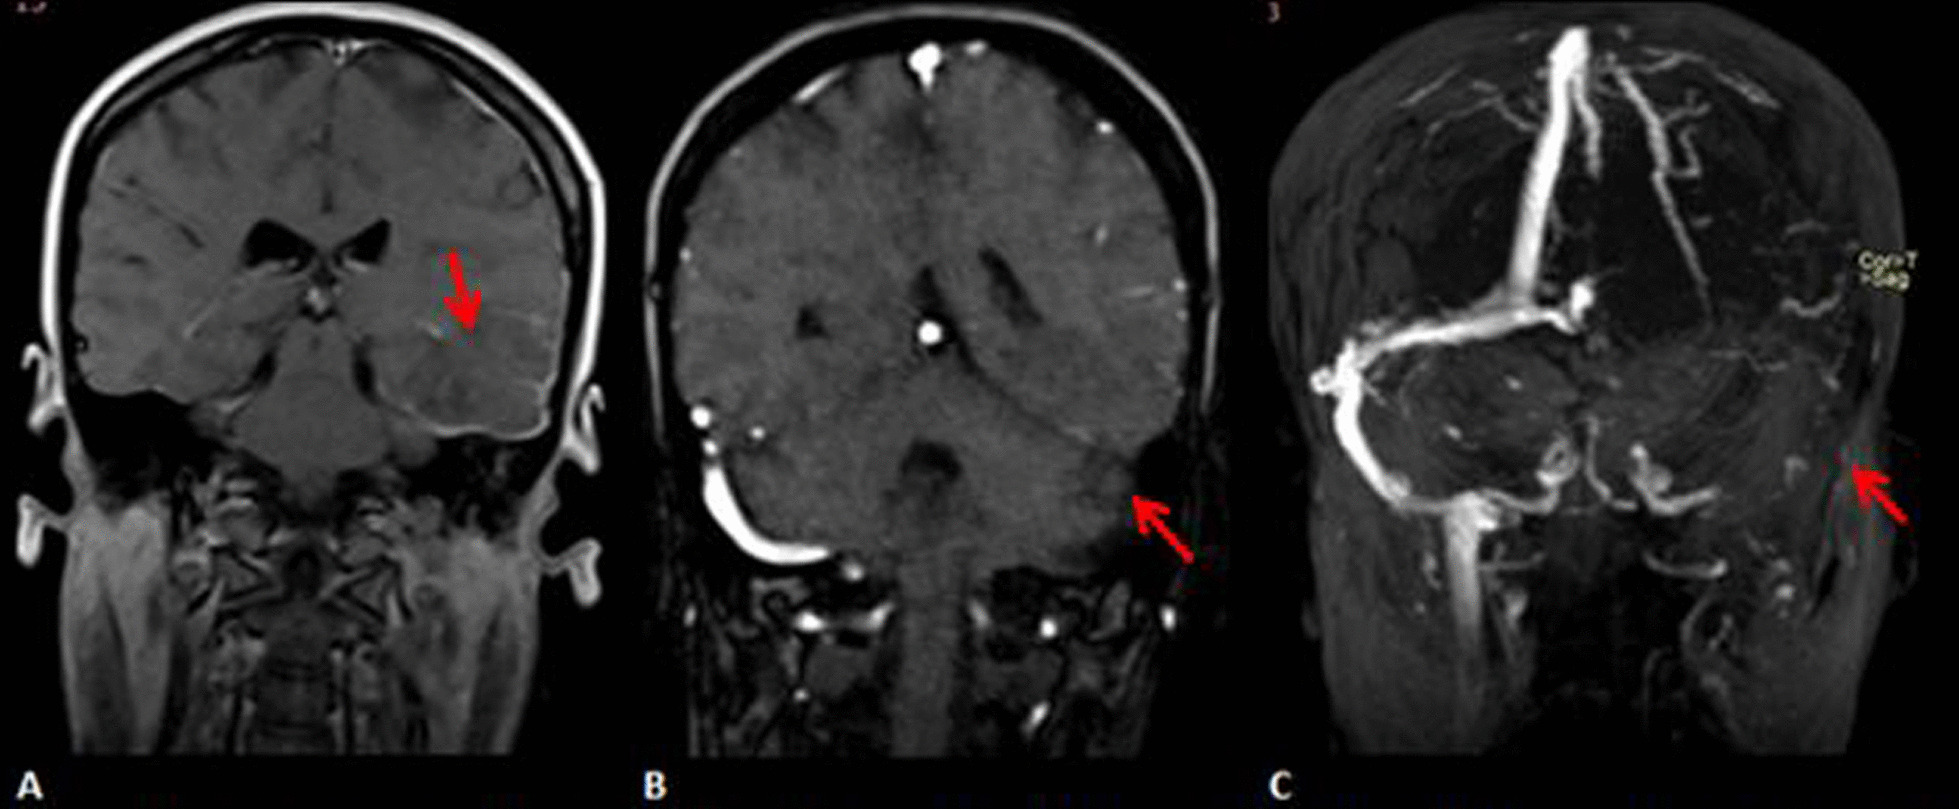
A Coronal T1 magnetic resonance imaging showing hypointense lesion in the left inferior parietal region (red arrow). Coronal (B) and 3-D (C) magnetic resonance venography showing filling defect of the left transverse and sigmoid sinuses along with corresponding cortical venues (red arrow)
